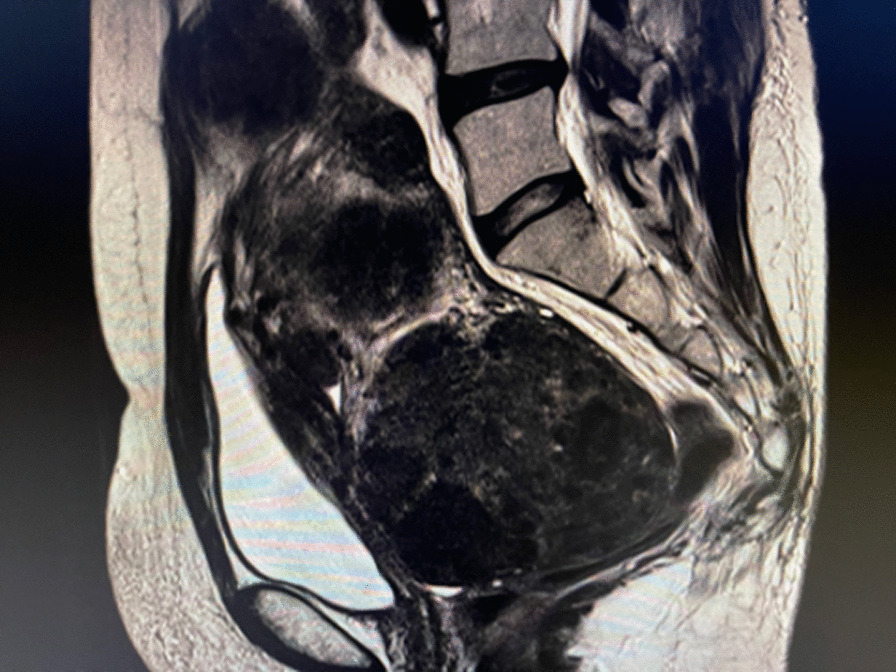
Magnetic resonance images showing a significant reduction of the uterus after 6 months of oral relugolix (40 mg/day). After relugolix therapy for 6 months, diffused adenomyosis mostly disappeared compared to the baseline. It is noteworthy that the size of the uterus, including fibroid and adenomyosis, shrunken to 15 cm in length and 8 cm in thickness
